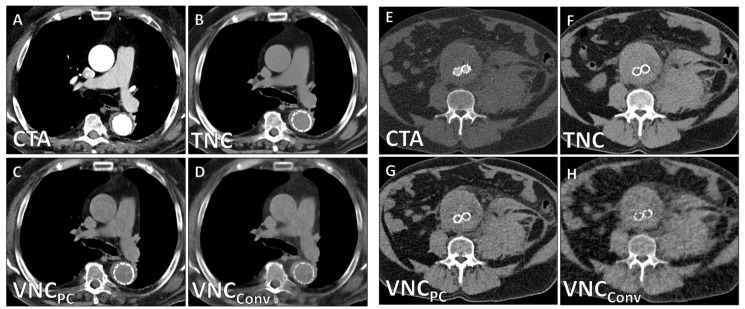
Representative PCD-CT images of a 72-year-old male patient after thoracic EVAR (A–D) and a 67-year-old male patient after abdominal EVAR (E–H).
